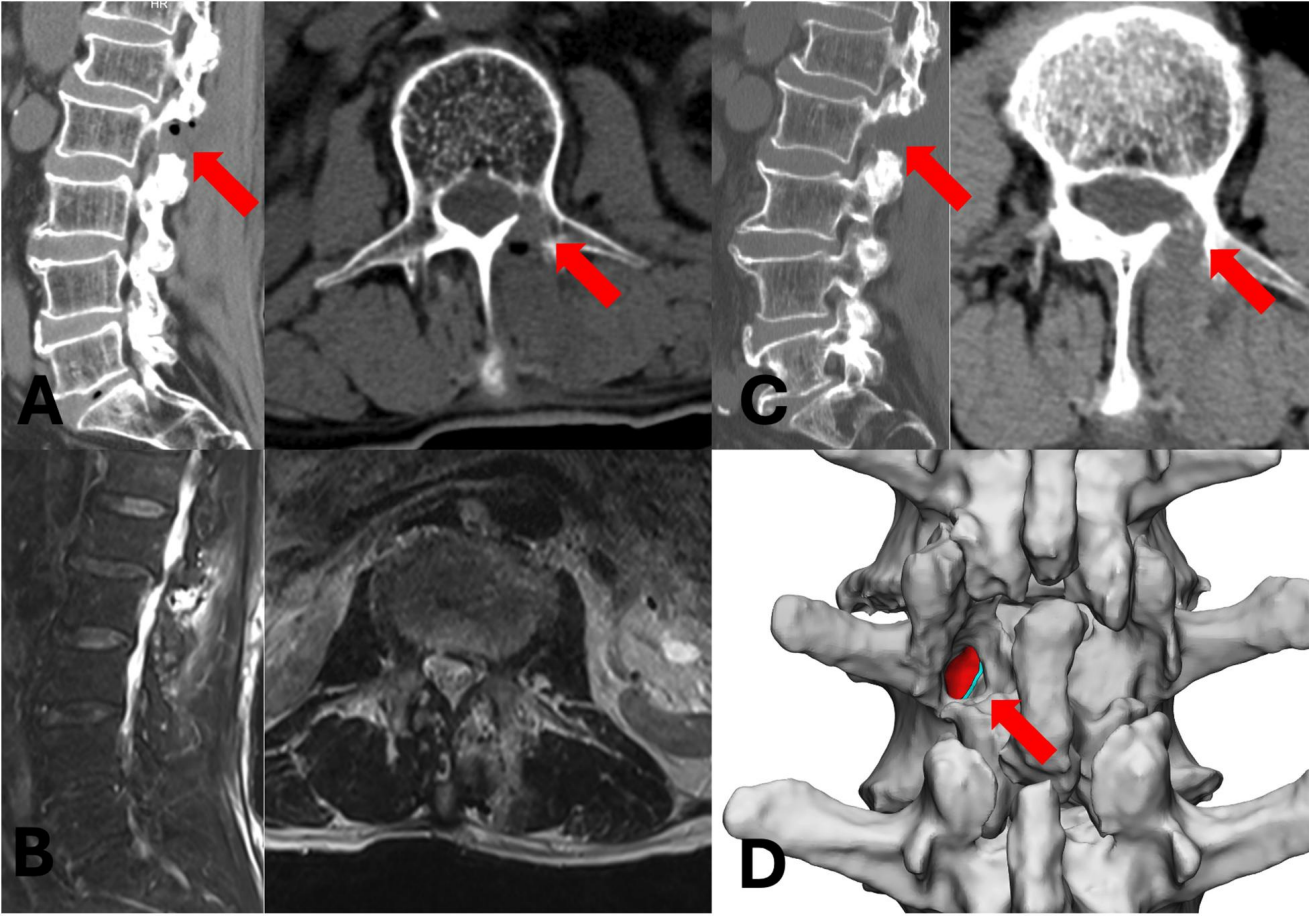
Postoperative radiological imaging. (A) Postoperative day 2 CT scan; the red arrow indicates the area of bone resection. (B) Postoperative day 3 MRI showing complete removal of the sequestrated disc. (C) Follow-up CT scan at 6 months postoperatively; the red arrow indicates the area of bone resection. (D) Three-dimensional (3D) CT reconstruction on postoperative day 2. The red area represents the cancellous bone of the pedicle, the blue strip-like area represents the medial cortex of the pedicle, and the red arrow indicates the extent of intraoperative bony decompression.
